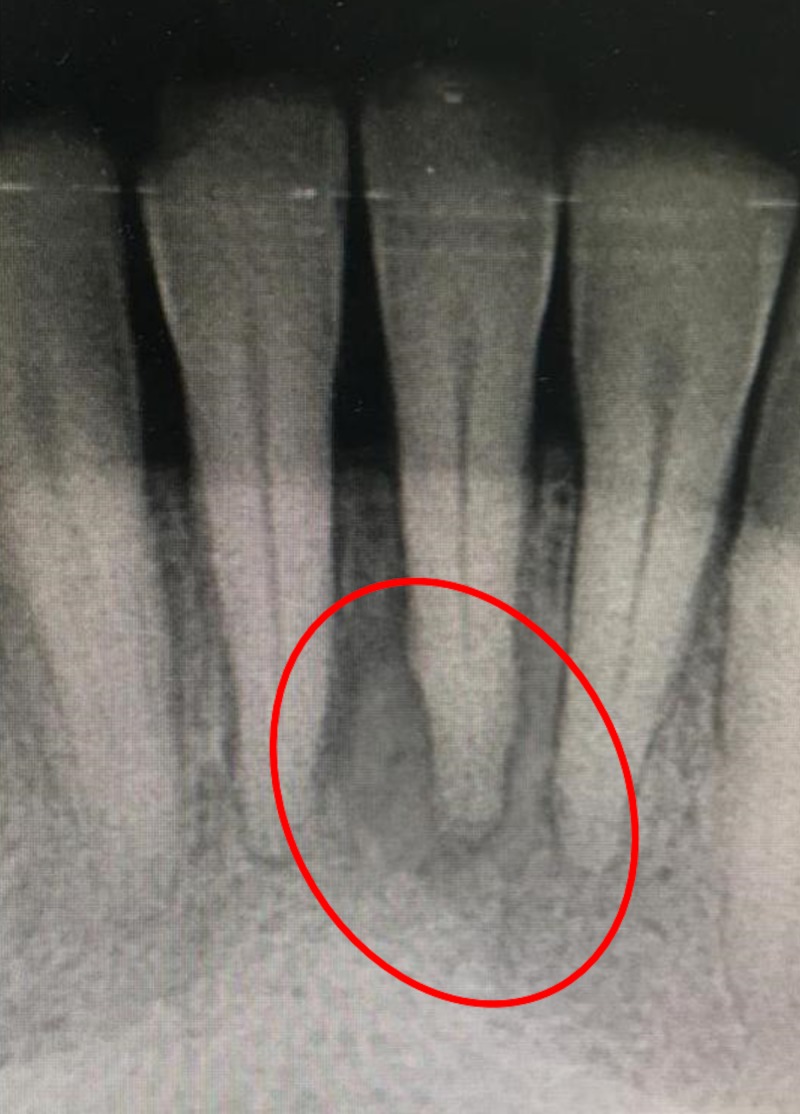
Periapical radiograph at three months follow-up.
